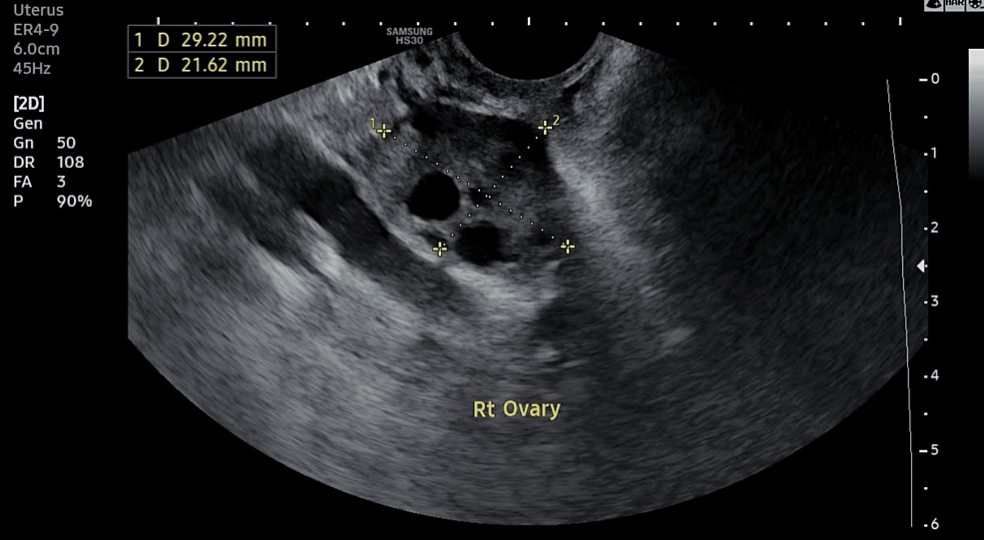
Right (Rt) ovary showing five follicles on day 2 via transvaginal sonography
